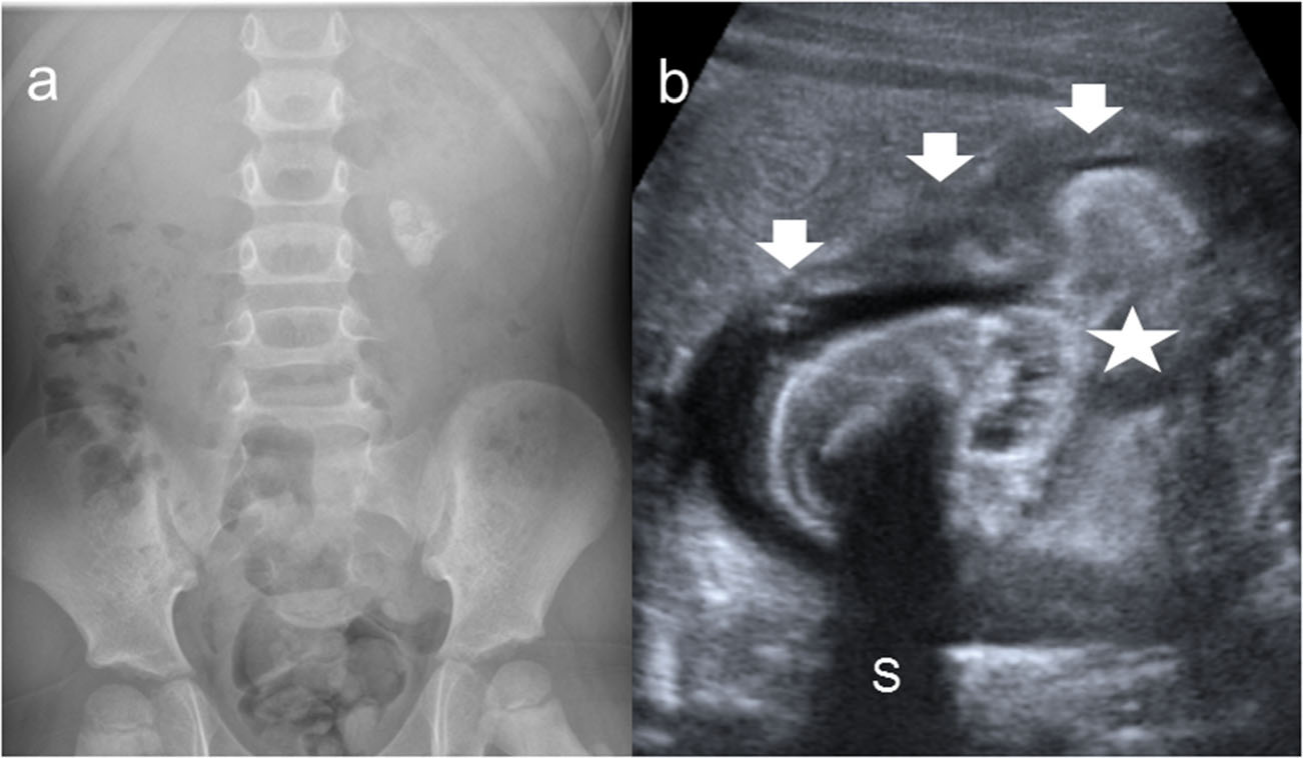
Diffuse XPN. a Plain X-ray of the abdomen showing a left staghorn calculus. b Transverse sonogram of the left kidney presents a stone with acoustic shadow (*S*) in a pelvis with important inflammatory and fatty component (*star*). Loss of parenchyma (*thick arrows*) is significant
